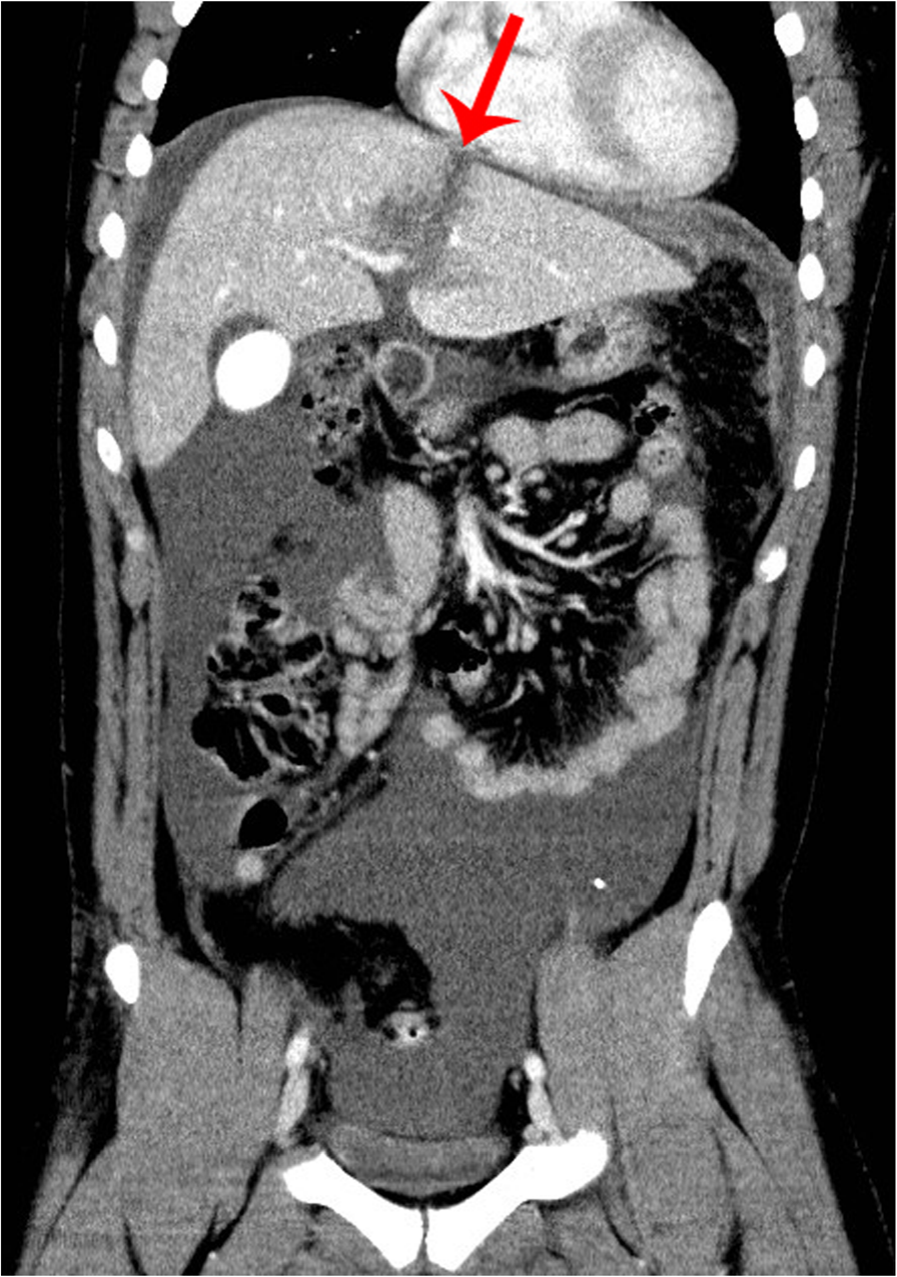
Following clinical deterioration, a repeated computed tomography (CT) scan demonstrated a localized grade 3 liver injury again and significant increase of free fluid in the abdominal cavity. The intended resection line was already outlined by traumatic rupture on the left side of the falciform ligament and indicated by arrow
